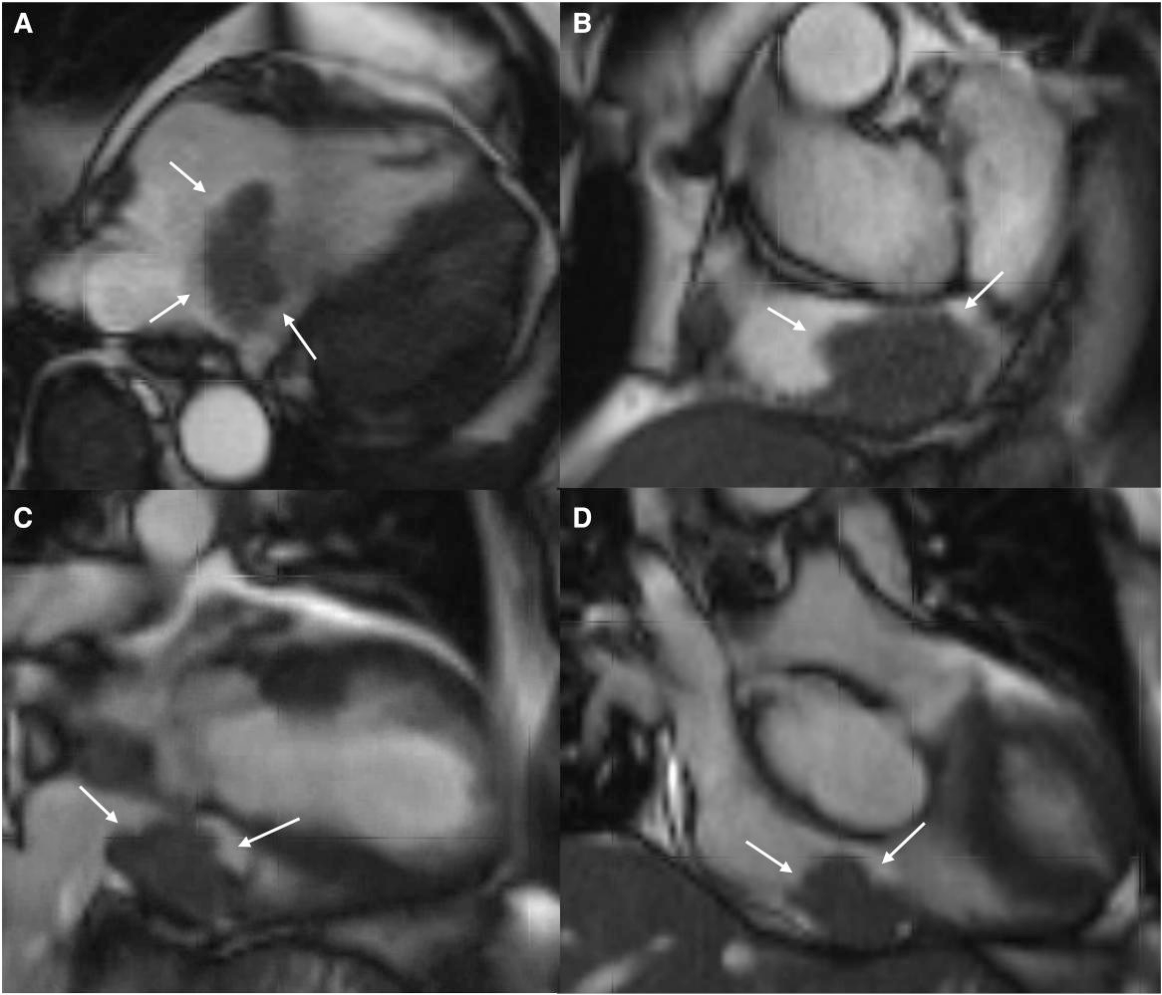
Cardiac magnetic resonance images with a steady-state coherent sequence with balanced gradients demonstrates the cardiac mass in the area of the right atrium (arrows) in (*A*) transversal imaging view, (*B*) short-axis view, (*C*) sagittal view, and (*D*) frontal view.
